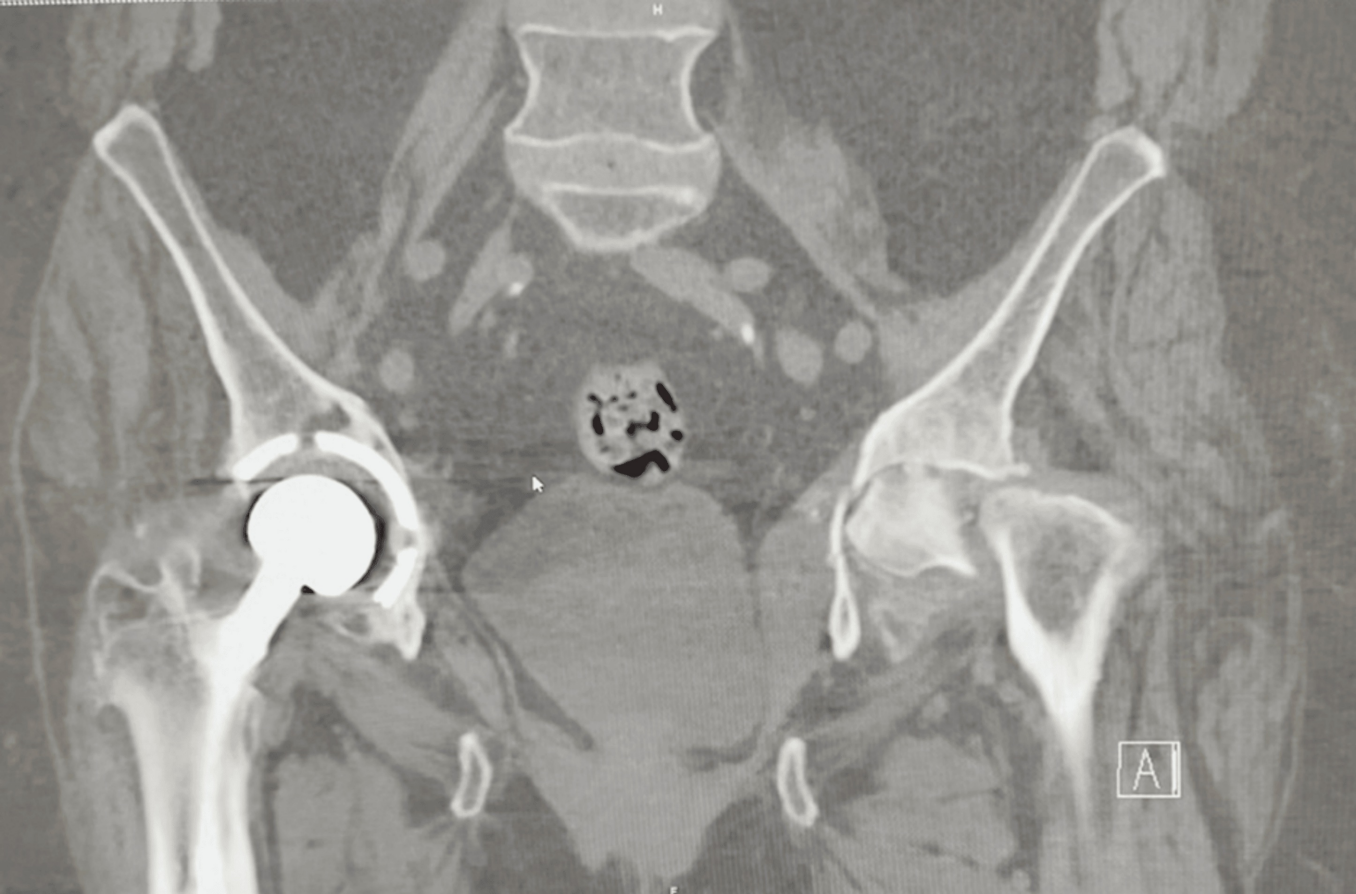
Additional preoperative coronal CT of the pelvis
